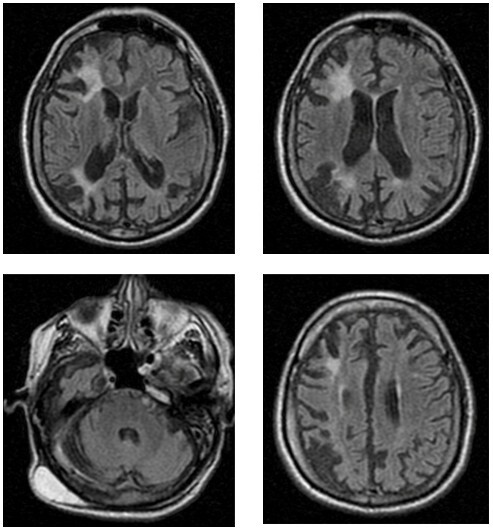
Axial Fluid-Attenuated Inversion Recovery — FLAIR-weighted MRI images demonstrating multiple hyperintense lesions involving both cortical and subcortical regions, predominantly affecting the frontal and parietal lobes, with associated deep and periventricular white matter involvement, consistent with chronic ischemic injury. In the posterior fossa, an axial FLAIR image reveals a hyperintense lesion in the right cerebellar hemisphere, compatible with a chronic ischemic infarct. Overall, the imaging pattern suggests a multifocal, chronic ischemic process involving multiple vascular territories, affecting both supratentorial and infratentorial structures.
